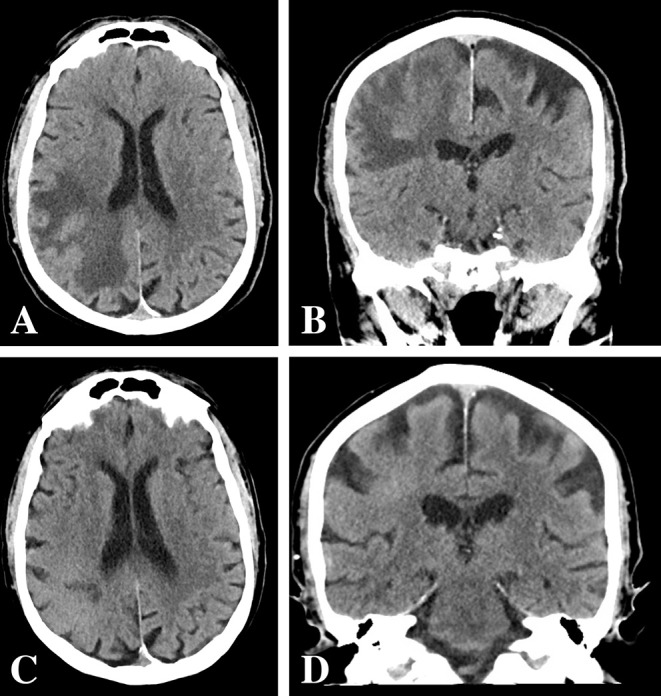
CT head pre- and post-corticosteroid therapy. (A,B) Initial CT head pre-corticosteroid therapy revealed a large hypodense lesion in the right parietal lobe with vasogenic edema. (C,D) Repeat CT head 4 weeks after post-corticosteroid therapy revealed a decrease in vasogenic edema and apparent size of the lesion.
